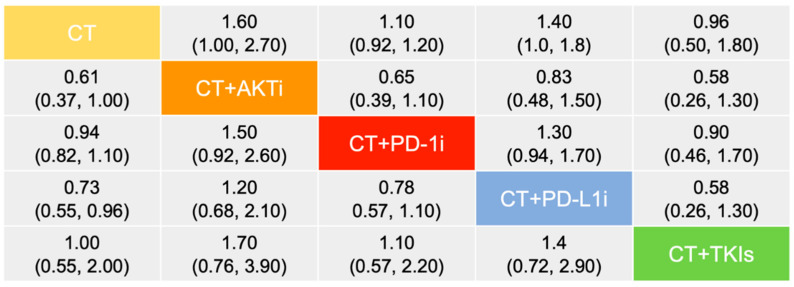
Comparative analysis of overall survival among adopted treatments. Data are presented as hazard ratio and 95% credible interval. The upper right values display the top treatment compared the bottom treatment. The lower left values demonstrate the converse comparisons. Abbreviation: AKTi, Protein kinase B inhibitor; CT, Chemotherapy; PD-1i, Programmed cell death protein 1 inhibitor; PD-L1i, Programmed cell death ligand 1 inhibitor; TKIs, Tyrosine kinase inhibitors.
